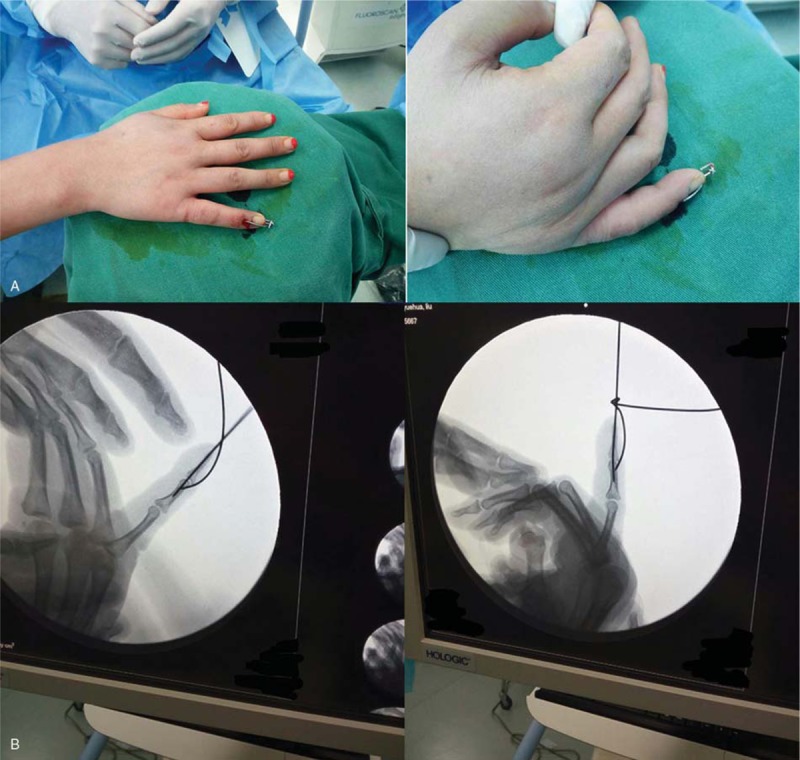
A: Photographs of the finger post-operation. B: X-ray images of the finger post-operation.
